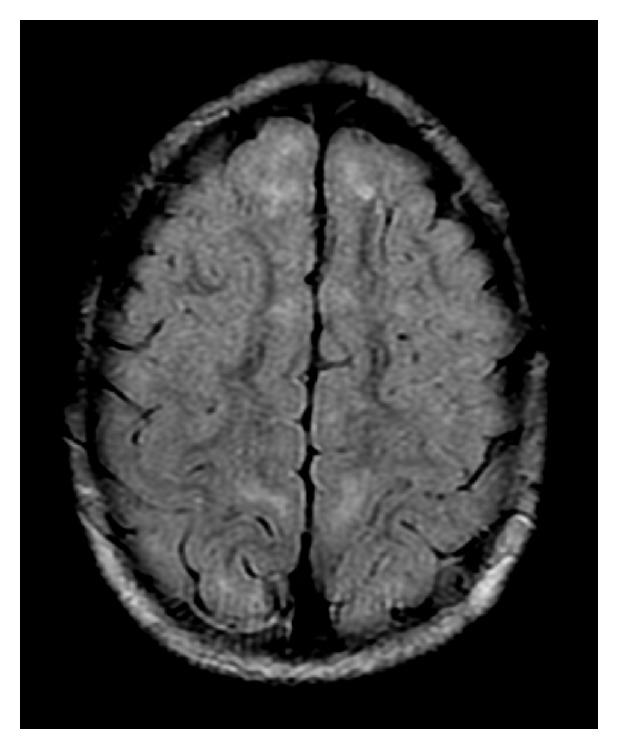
Axial T2-FLAIR MRI image demonstrating subcortical white matter hyperintensities most prominent within the frontal lobes. Image is courtesy of Dr. Jane Congeni.
